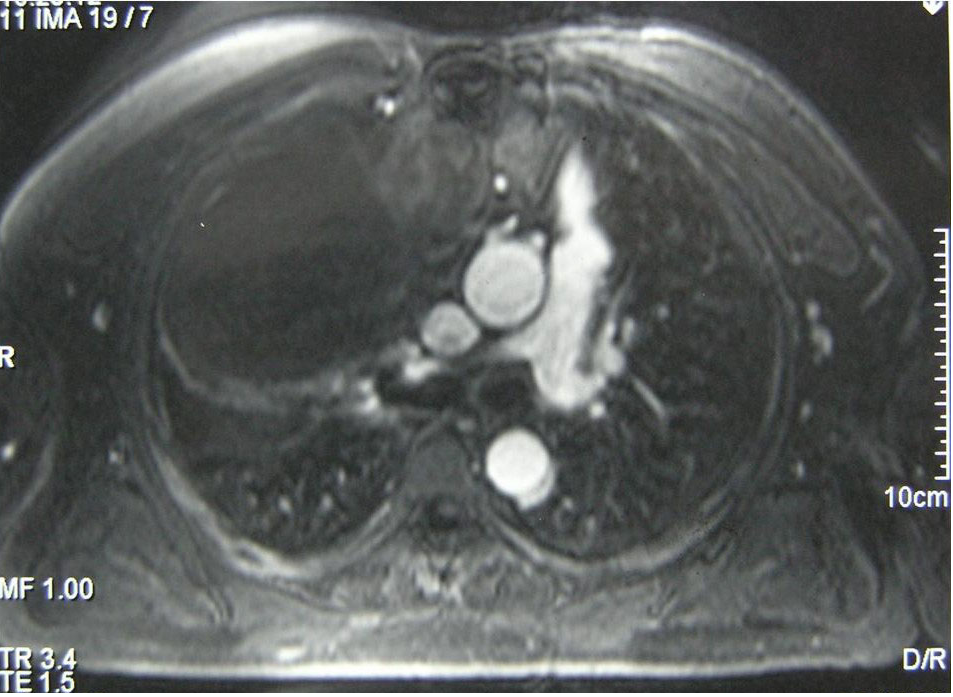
MRI transverse scan showing mass extension and anatomical relationship between the tumour and the tree grafts at level of ascending aorta.
